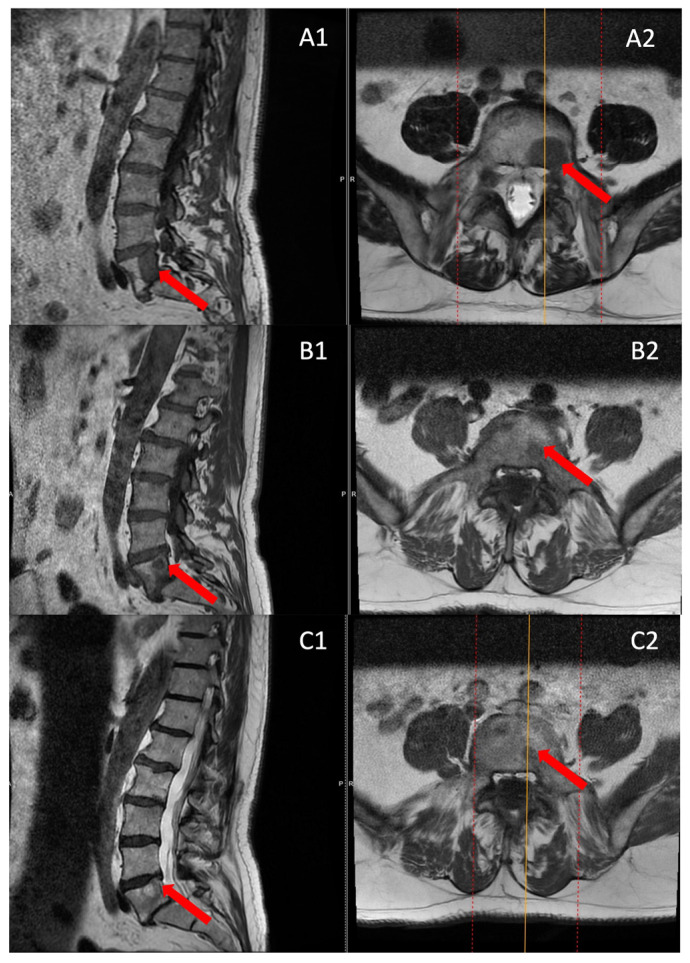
MRI scans before surgery (A), 6 months post-surgery (B), and 12 months post-surgery (C) were examined in both sagittal (A1,B1,C1) and axial sections (A2,B2,C2). The red arrow shows the lesion location.
